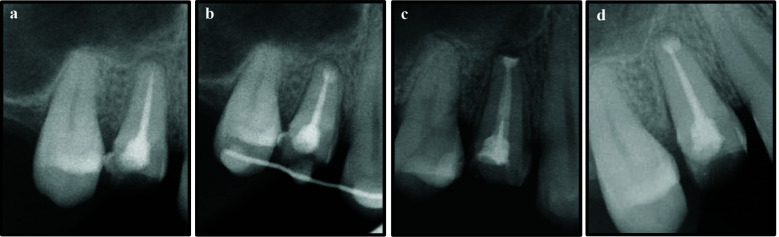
a, Preoperative radiograph showing previously treated tooth 14; b, Immediate post intentional replantation and splinting of tooth 14; c & d, Three months and six months follow-up respectively.
